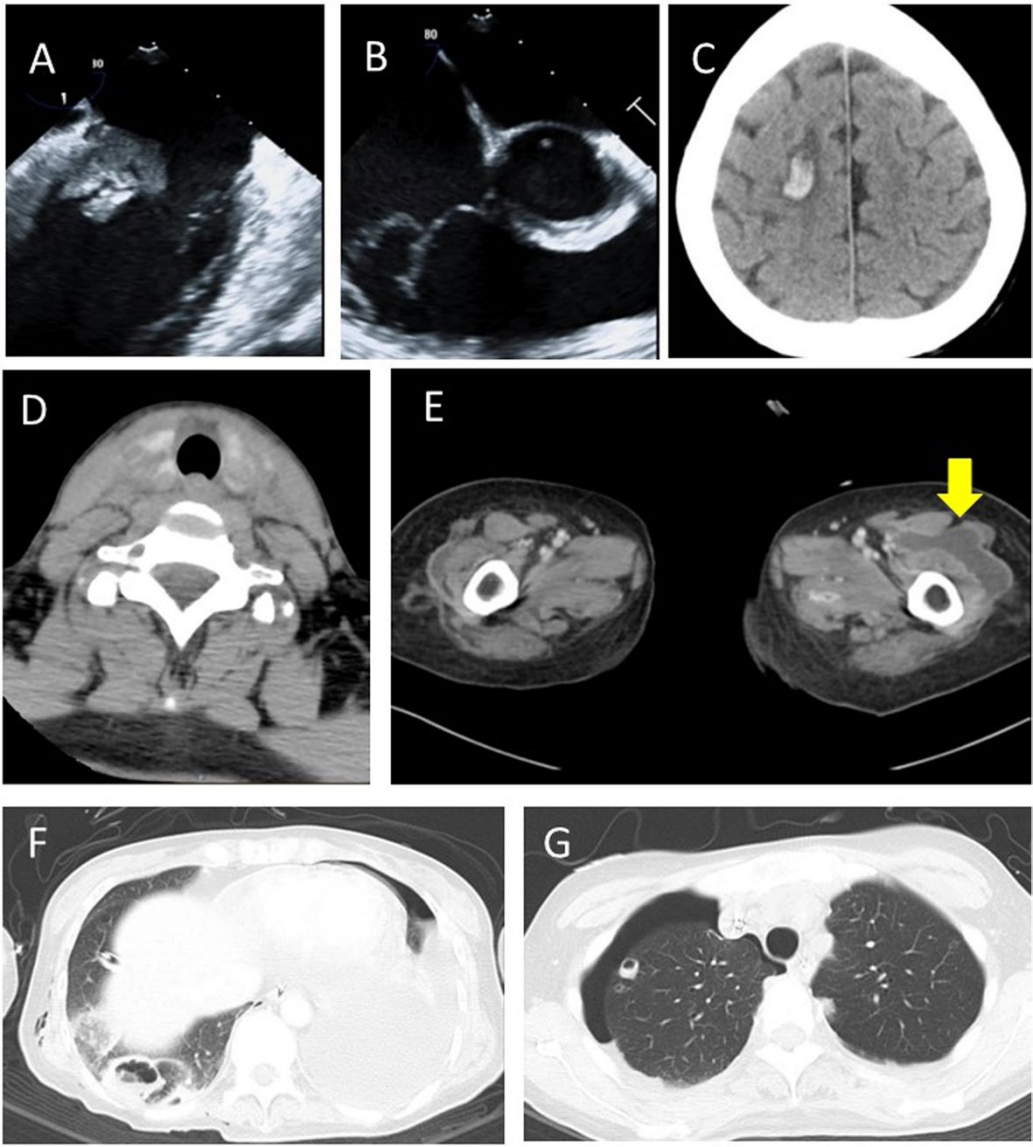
A 22 × 10 mm mobile mass on the posterior leaflet of the mitral valve (transesophageal echocardiography [TEE]). B Mobile mass on the posterior leaflet and the chordae tendineae of the tricuspid valve (TEE). C Hemorrhagic cerebral infarction (Brain computed tomography [CT]). D Bilateral thyroiditis (contrast-enhanced CT). E Abscess in the left thigh (contrast-enhanced CT). F Abscess in the right lung (chest CT). G Secondary pneumothorax in the right lung (chest CT)
